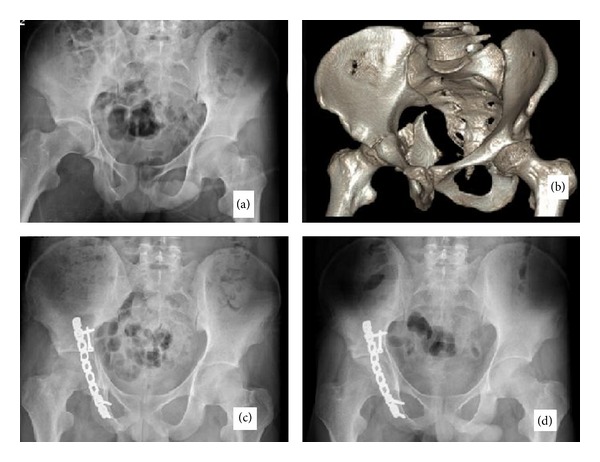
A 26-year-old male patient having acetabular fracture. (a) Preoperative X-Ray. (b) Preoperative 3D-CT scan. (c) Early postoperative X-Ray. (d) Late postoperative X-Ray (24 months).
